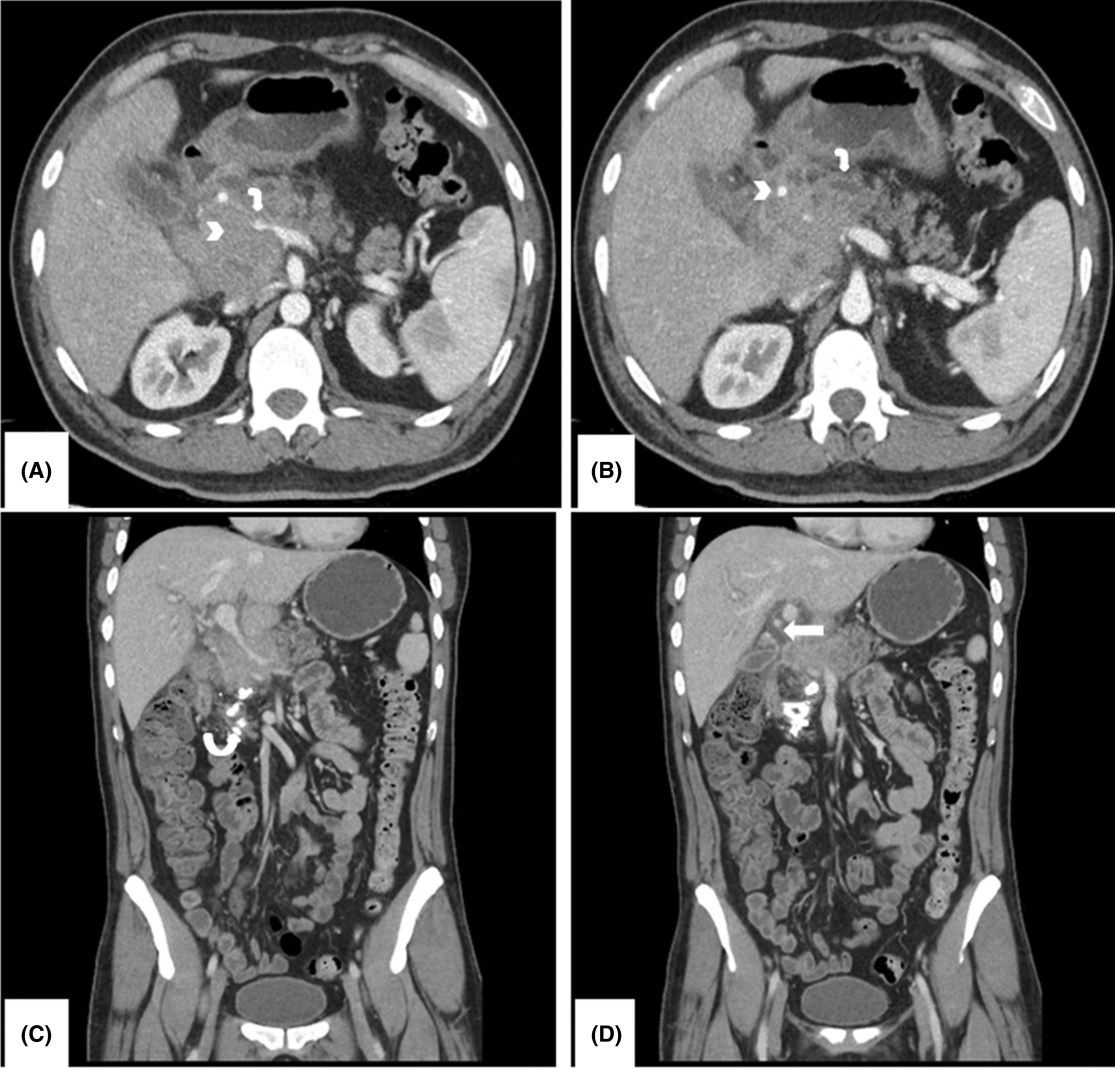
A 39‐year‐old male CT abdomen examination was requested with a history of recurrent pancreatitis. Serum IgG4 levels were elevated to 2960 mg/L. (A) Axial section from abdominal CECT showing a mildly enhancing but heterogeneous, soft tissue density mass involving the head of the pancreas (white arrowhead), which is completely encasing the portal vein resulting in its narrowing (bent arrow). (B) Axial section from CECT showing that mass is encasing the hepatic artery properly (white arrowhead). There is mild dilatation of the pancreatic duct measuring at 3.5 mm (bent arrow). (C) Coronal section from CECT again showing significant portal vein narrowing without thrombosis. Foci of coarse calcification is seen in the head and uncinate process, in keeping with chronic pancreatitis (circular arrow). (D) Coronal section from CECT showing faint enhancement of the common hepatic duct, one of the ancillary findings seen with IgG4‐related disease (white arrow).
